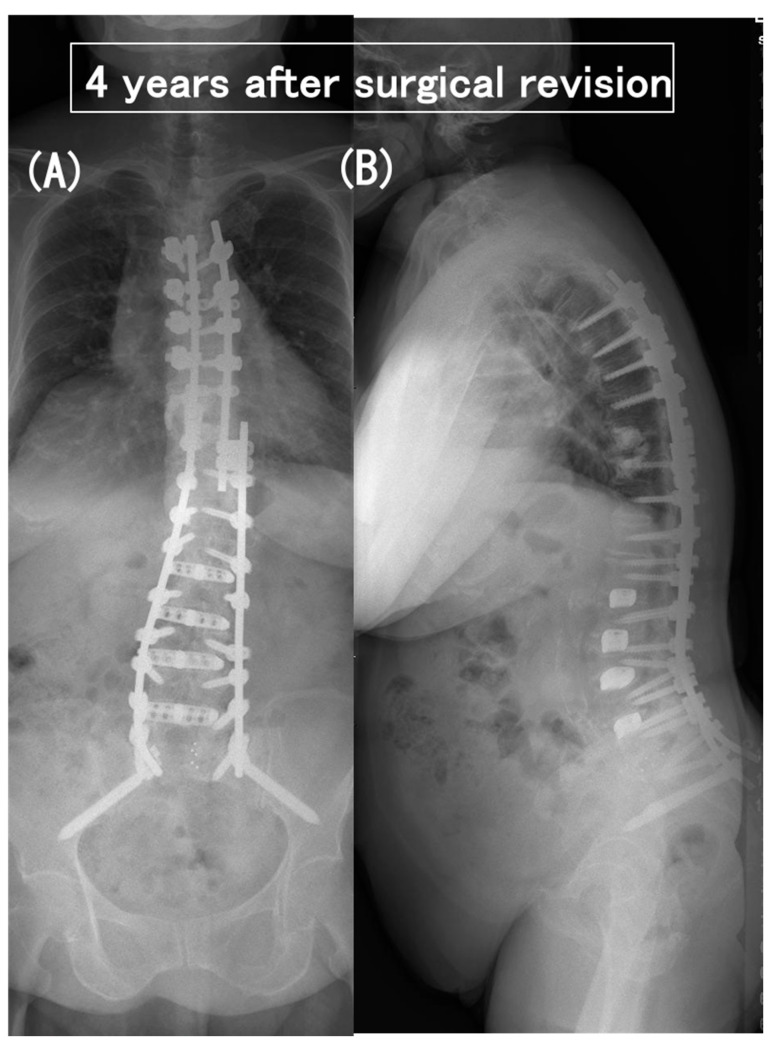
Coronal (A) and sagittal (B) full-length standing radiographs obtained 4 years after surgical revision. The paraparesis gradually improved from modified Frankel grade C to D2.
